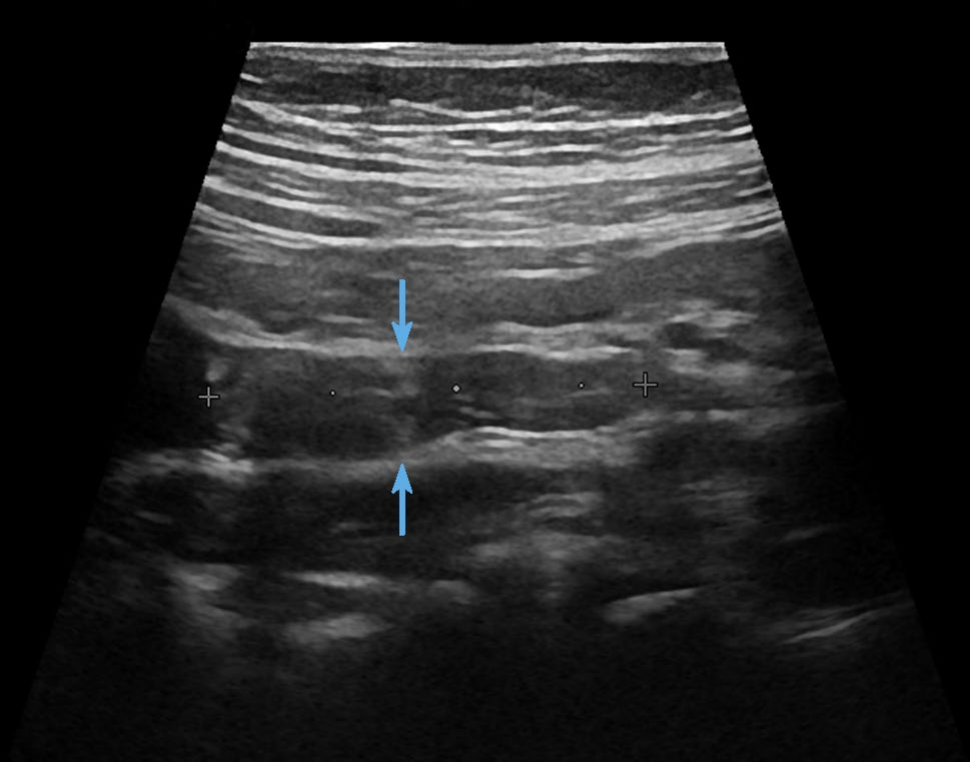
11-Year-old boy with intermittent abdominal pain, longitudinal sonogram reveals
